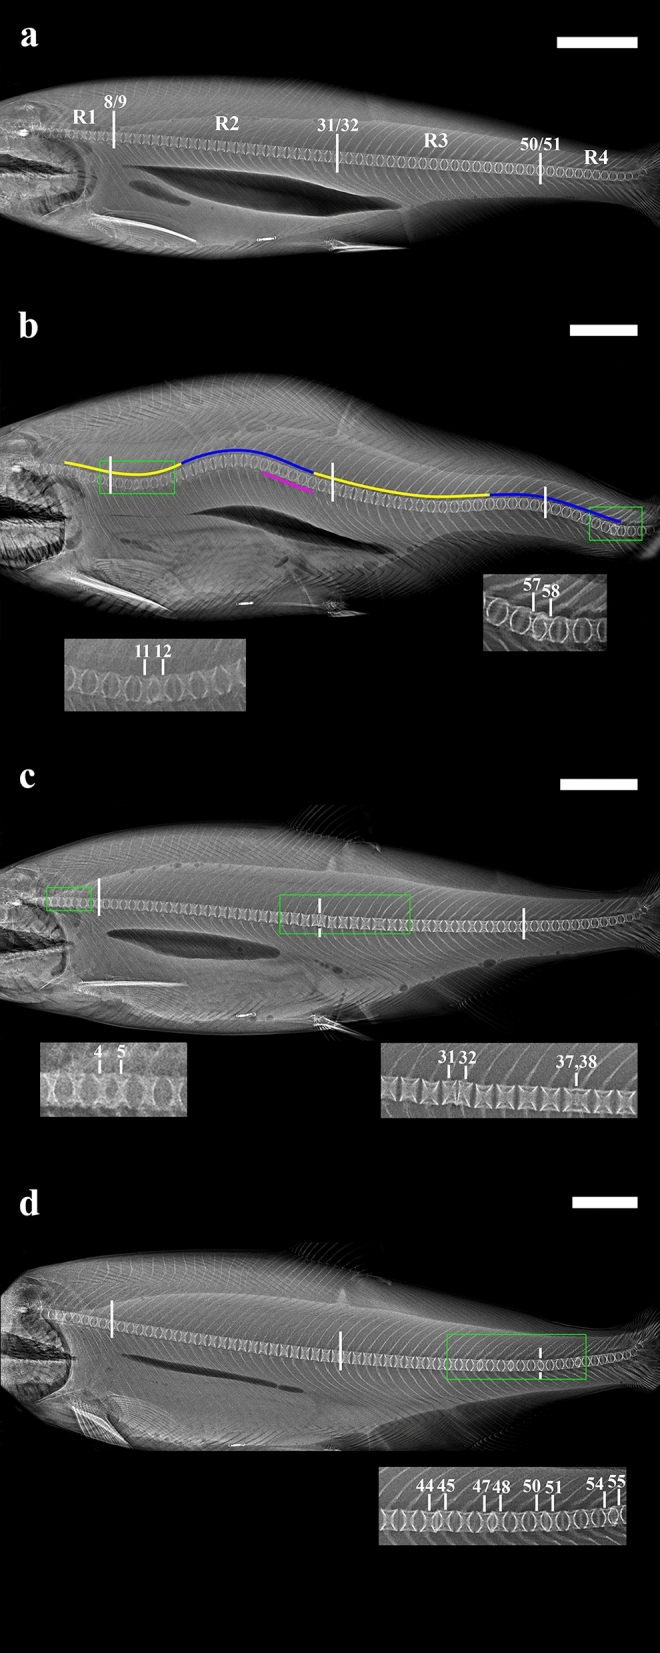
Representative examples of main spinal anomaly types (based on Witten et al*.*^15^) assessed on lateral radiographs of farmed NZ Chinook salmon 13–15 months post-seawater transfer. Labels R1, R2, R3 and R4 in (a) correspond to spinal regions 1 (vertebra (V)1–8), 2 (V9–31), 3 (V32–50) and 4 (V51–62+). Small vertical white lines indicate the transition points between each of the four spinal regions. Inset images are included where vertebral body anomalies (vertebral compression, fusion and vertical shift) are present and correspond to the location of green boxes on main images. Numbers in insets represent vertebra numbers. Scale bars = 5 cm. (a) No anomaly detected (NAD). All vertebrae exhibit the regular “X in a box shape”, are evenly spaced, and are dorso-ventrally aligned with one another in all regions. The vertebral column exhibits no angular deviation indicative of spinal curvature. (b) Spinal curvature (Types 14–16^15^). Lordosis (curvature towards the ventral surface, Type 14^15^), is indicated in yellow, kyphosis (curvature towards the dorsal surface, Type 15^15^) in blue, and presumed scoliosis (lateral curvature discernible on lateral radiographs as change in vertebral shape from “X in a box” to biconcave, Type 15^15^) in pink. Vertebral compression as reduction in intervertebral space (Type 1^15^) and vertical shift (Type 17^15^) is also present at vertebrae (V)11–12 and V57–58. (c) Vertebral compression as reduced intervertebral space (Type 1^15^) at V4–5 and V31–32. V31–32 also exhibit one-sided compression (Type 5^15^). V37–38 are almost completely fused (Type 6 progressing to Type 7^15^). (d) Vertebral compression as reduction in intervertebral space (Type 1^15^) and vertical shift (Type 17^15^) present at V44–45, V47–48, V50–51 and V54–55.
